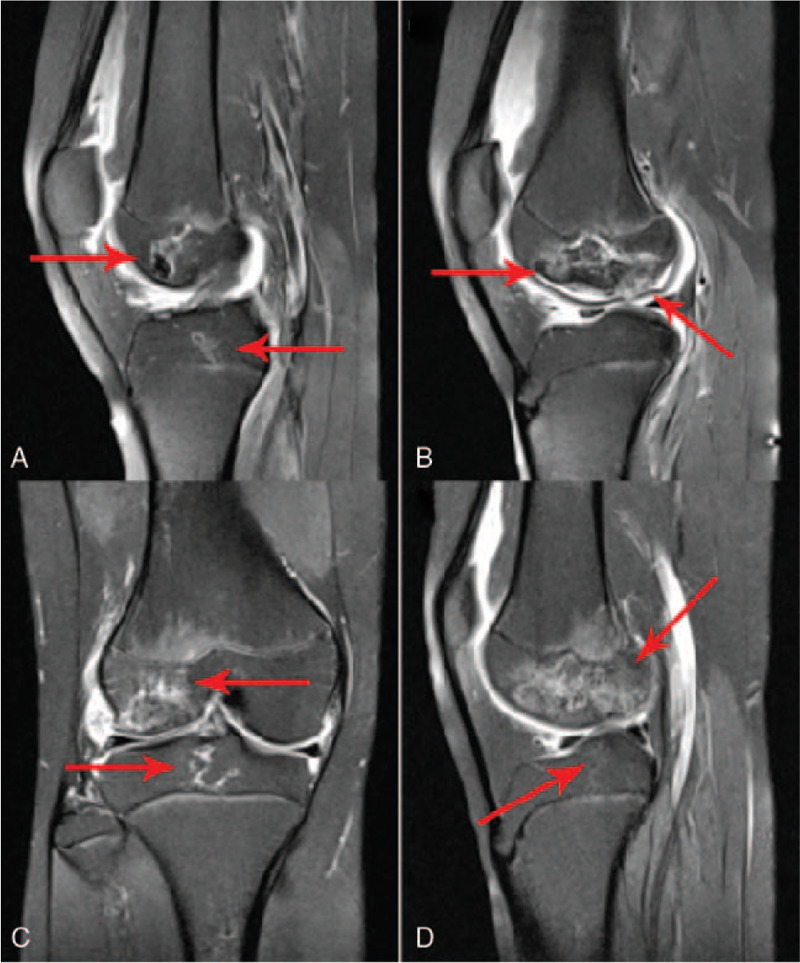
Bilateral knee joint magnetic resonance imaging appearance of the systemic lupus erythematosus patient with osteonecrosis (A) and (B) Sagittal magnetic resonance imagings of left knee joint. (C) and (D) Sagittal magnetic resonance imaging of right knee joint. The figure demonstrated irregular bone destruction and bone hyperplasia lesions on bilateral distal femur and proximal tibia, presenting geographic alterations.
